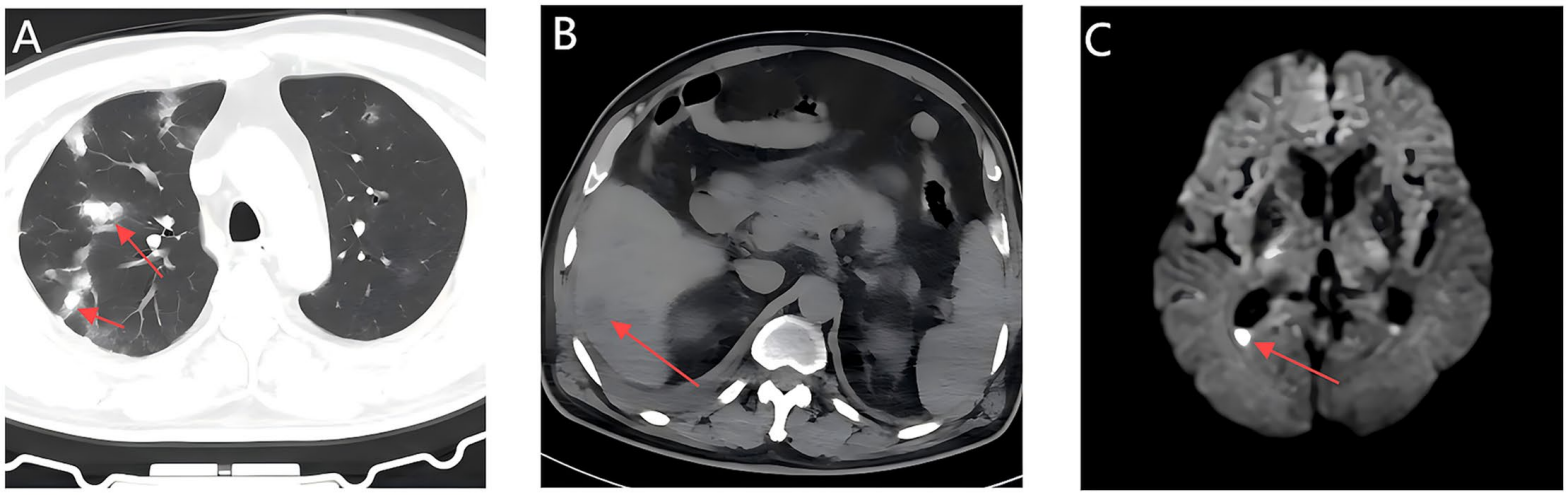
Initial multimodal imaging findings within 24 hours of admission; Chest CT, Abdominal CT, and Brain MRI. (A) A Chest CT scan shows multiple patchy hyperdense opacities in bilateral lungs. (B) An abdominal CT scan shows hypodense lesion in the right posterior hepatic lobe (arrow). (C) Axial MRI head shows mild enlargement of the supratentorial ventricular system, with fluid levels in the occipital horn of the lateral ventricle accompanied by hyperintensity on DWI (arrows).
